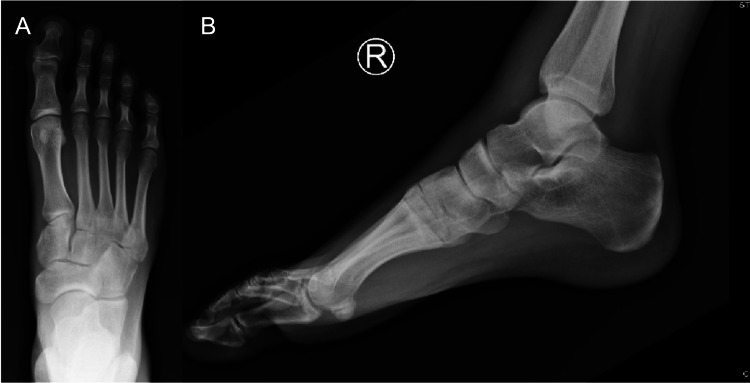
Anterior/posterior (A) and lateral (B) radiograph view of the right foot showing no evidence of fracture, gas, radiolucency, or opacity.
